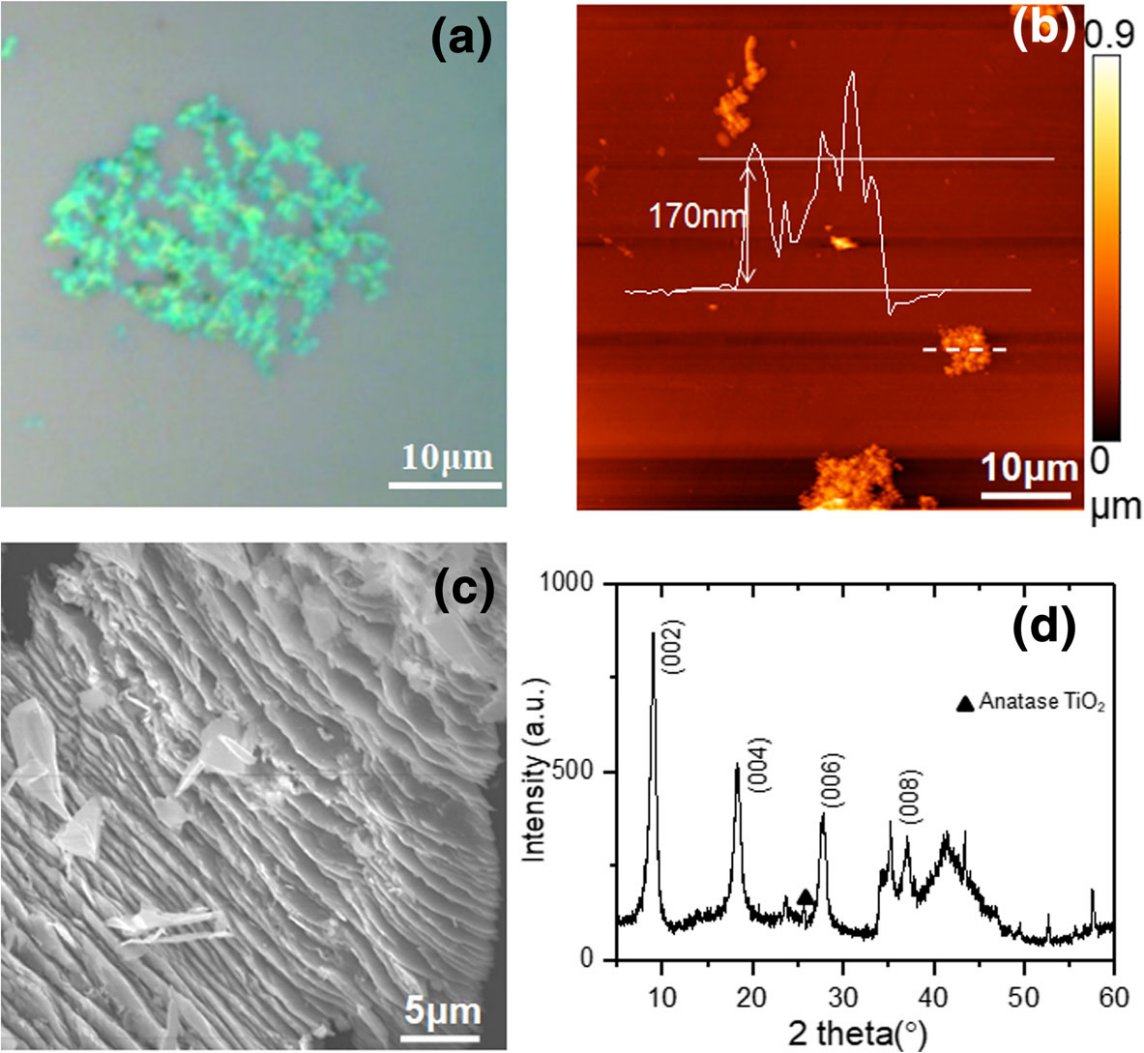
a Optical image of ultrasonically exfoliated Ti_3_C_2_T_*x*_ flakes; b AFM topographic image of ultrasonically exfoliated Ti_3_C_2_T_*x*_ flakes, and a line profile across the marked dashed line is shown as an inset, indicating the Ti_3_C_2_T_*x*_ flake thickness of 170 nm; c SEM image of ultrasonically exfoliated Ti_3_C_2_T_*x*_ flakes; d XRD spectra of Ti_3_C_2_T_*x*_ raw powder
